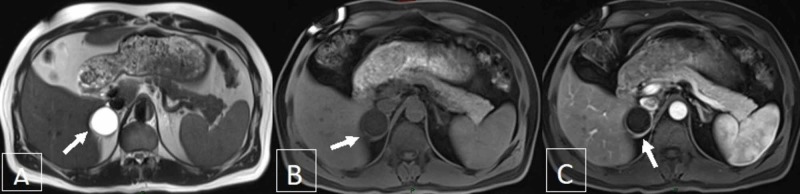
MRI of the abdomen and pelvis with gadolinium (A) right adrenal cystic lymphangioma lesion (arrow) as evident on T2 sequence, axial view, measuring around 4.0 x 3.7 cm with homogeneous signal intensity; (B & C) T1 sequence, pre- and post-contrast phase, respectively, showing the same cystic lymphangioma lesions, arising from the right adrenal gland, exhibiting rim enhancement (arrow) with absence of solid or fat component MRI, magnetic resonance imaging
